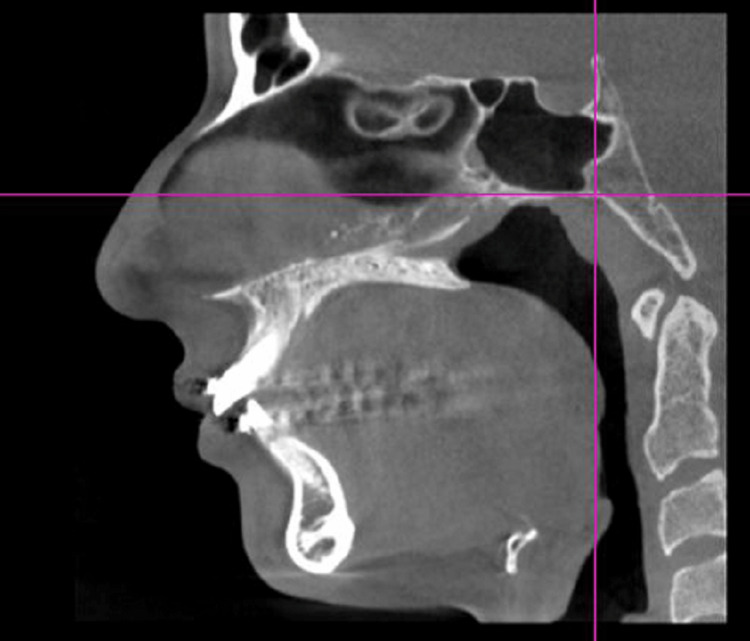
CBCT pre-treatment with Forsus CBCT - Cone beam computed tomography
